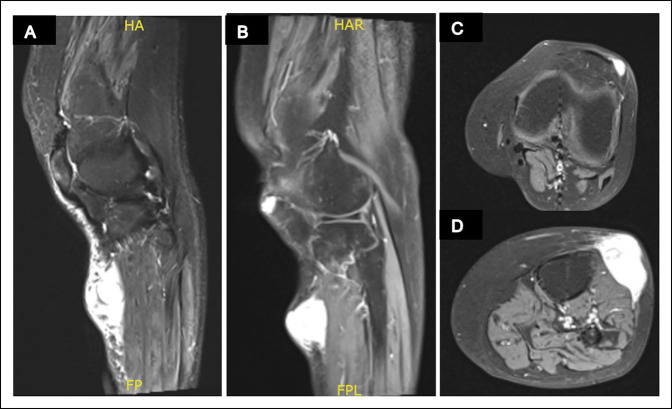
Radiographs showing MRI scans of the knee and proximal leg demonstrating both lesions. A, Sagittal T2 with hyperintensity indicative of the ovoid sarcomatous mass on the proximal anterolateral aspect of the leg. B, Sagittal fat-saturated T1 post-contrast demonstrating both the sarcomatous mass and a more proximal circumscribed hyperintensity about the anterolateral aspect of the patella which corresponded with the glomus tumor. Axial fat-saturated T1 post-contrast images (C) proximally at the level of the glomus tumor and (D) distal to the knee at the level of the soft-tissue sarcoma.
